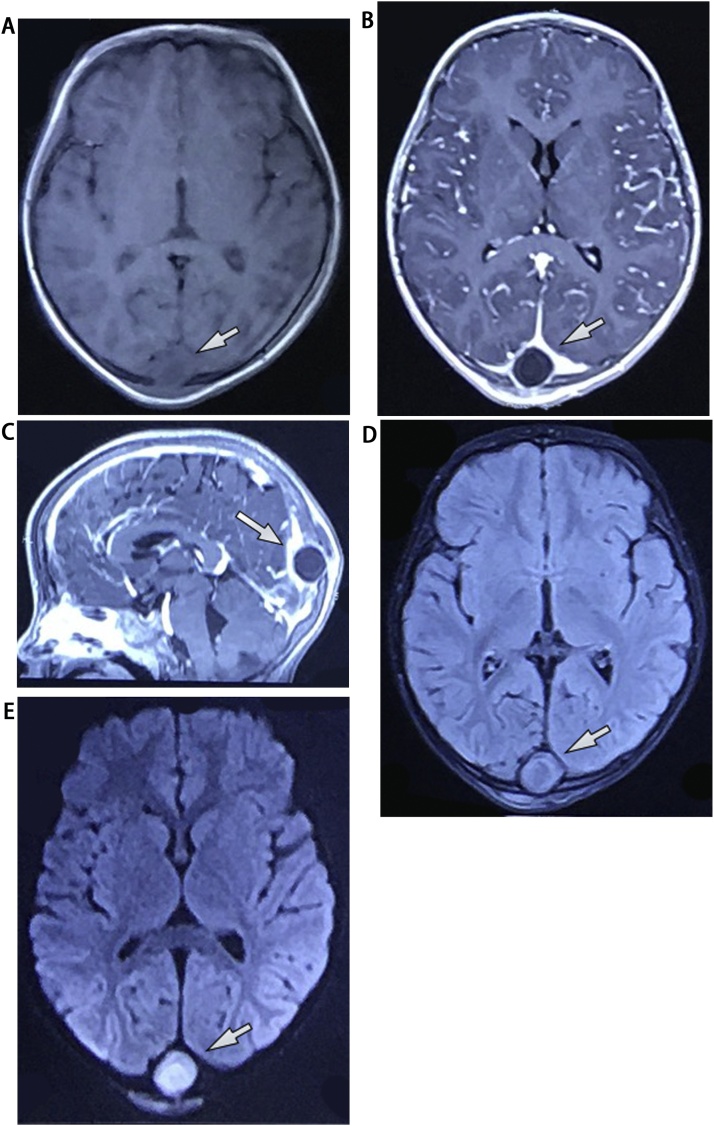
On preoperative MRI, an extra-axial isointense mass on T1W enhanced peripherally and invaded superior sagittal sinus on gadolinium-enhanced T1W, was hyperintense on FLAIR and hypoperfused on DWI.
